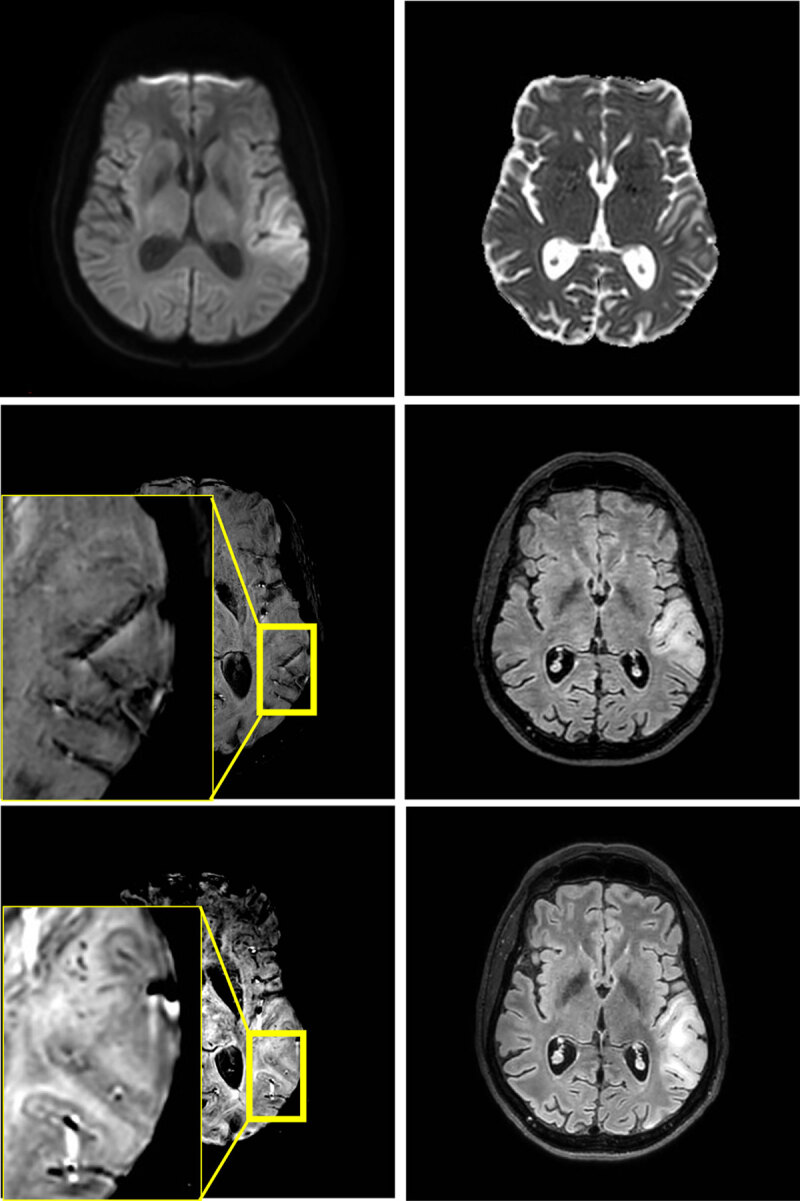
Edema in the left temporoparietal operculum without reduced diffusivity on MRI at 3T at first presentation (4 upper images). No microhemorrhages are found on the first scan, but they are present in juxtacortical location at one-week follow-up (2 lower images).
